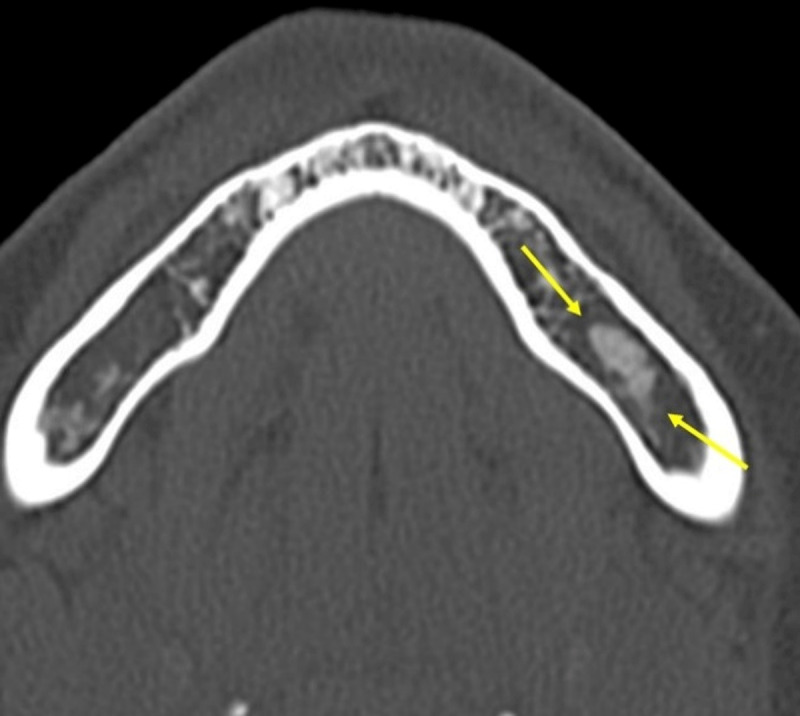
Idiopathic osteosclerosis. Axial CT showing a sclerotic lesion with somewhat spiculated margins (arrows). No low-attenuation rim is seen.
